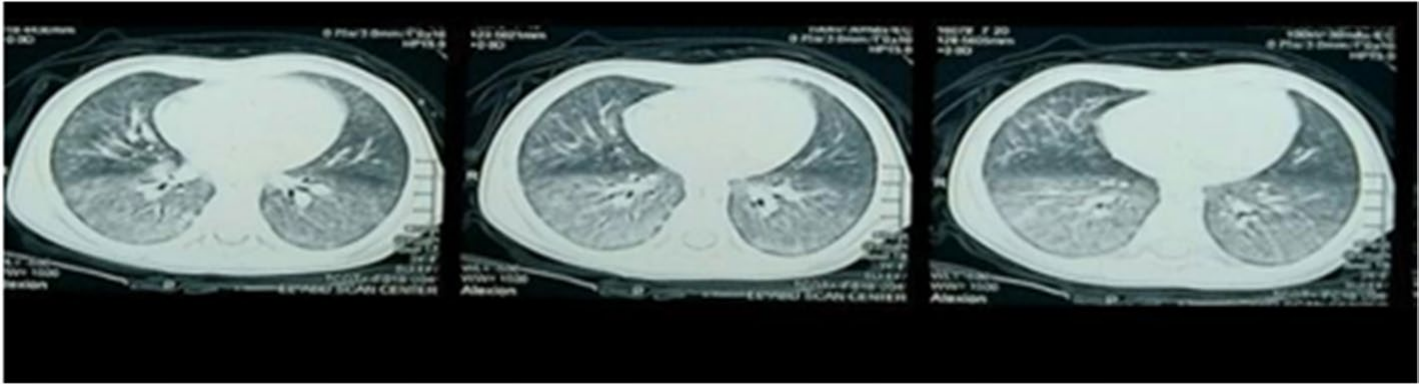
Bilateral lower lobar, to less extent middle lobar diffuse ground glass attenuation, reticulonodular densities, and peribronchial thickening (HRCT patient number 4)
